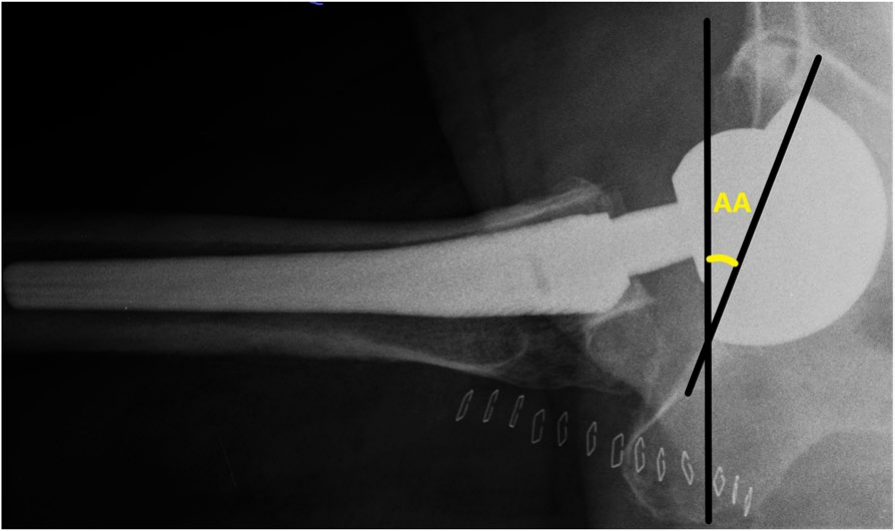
AA measurement. A case of THA with cross-table lateral radiograph for the measurement of AA. See text for the description of this angle
